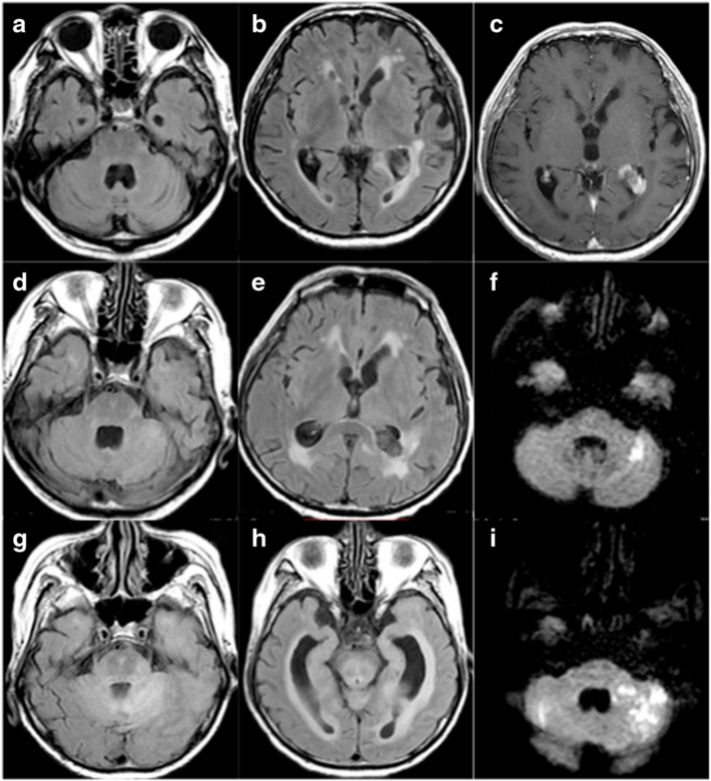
Brain MRI of the present patient at admission (a –c), and 17 days (d–f) and 40 days (g–i) after admission. Fluid attenuated inversion recovery (FLAIR) images at admission showed no gross abnormality, including in the brainstem and cranial nerves (a, b), although a mass-enhancing lesion was observed in the trigone of the left lateral ventricle on T1-weighted imaging (c). 17 days after admission, FLAIR images revealed slight hyperintensity in the exit for the trigeminal nerve and the left cerebellar hemisphere (d, e), and diffusion-weighted imaging (DWI) showed hyperintensity in the left cerebellar hemisphere (f). At the final MRI at 40 days after admission, diffuse high-intensity lesions and hydrocephalus were observed in the brainstem and cerebellum on FLAIR (g, h) and DWI revealed multiple hyperintense lesions in the bilateral cerebellar hemispheres (i)
